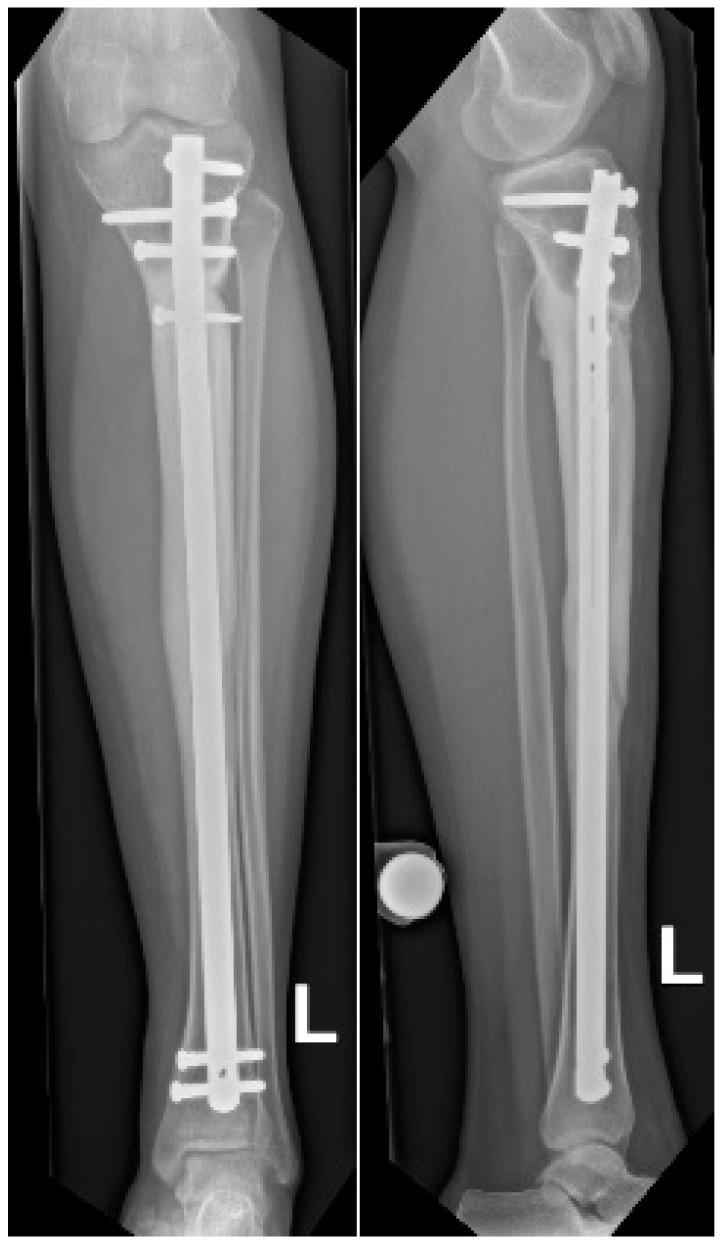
Radiographs at 18 months follow-up showing anteroposterior view (left) and lateral view (right) of the tibia. Consolidation can be observed, demonstrated by bridging callus across cortices and the absence of visible fracture lines, with restoration of tibial alignment.
